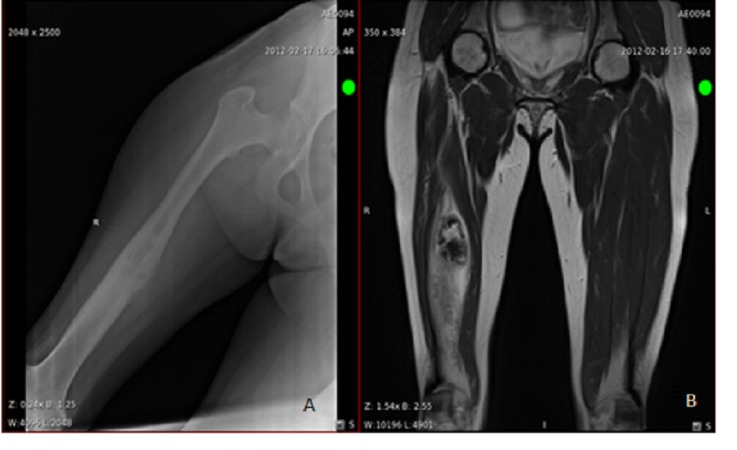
Radiological images of osteosarcoma. Right femur AP view (A), MRI without contrast coronal view (B).
